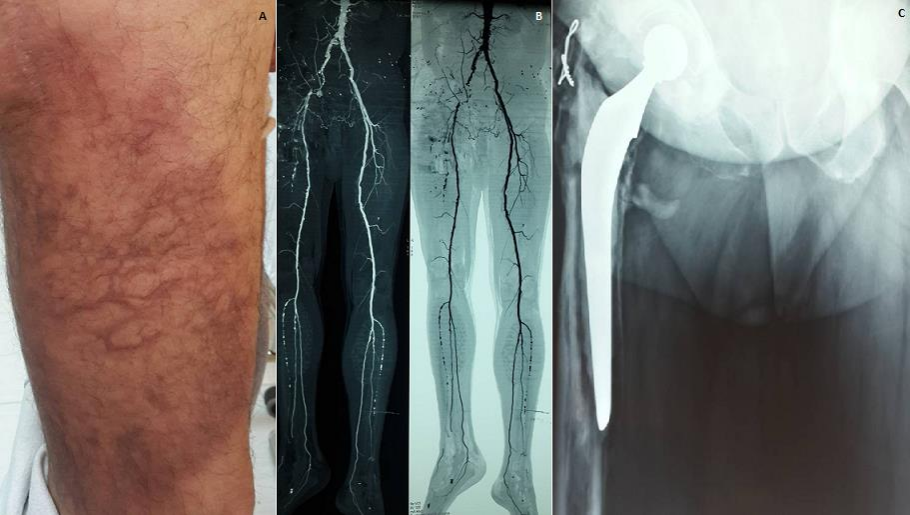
A) placard inflammatoire, écchymotique, avec livédo de la cuisse droite; B) radiographie standard de la hanche droite montrant un descellement de la prothèse totale de la hanche; C) angio-scanner montrant une occlusion de l’axe iliaque externe droit et une double sténose iliaque primitive droite
